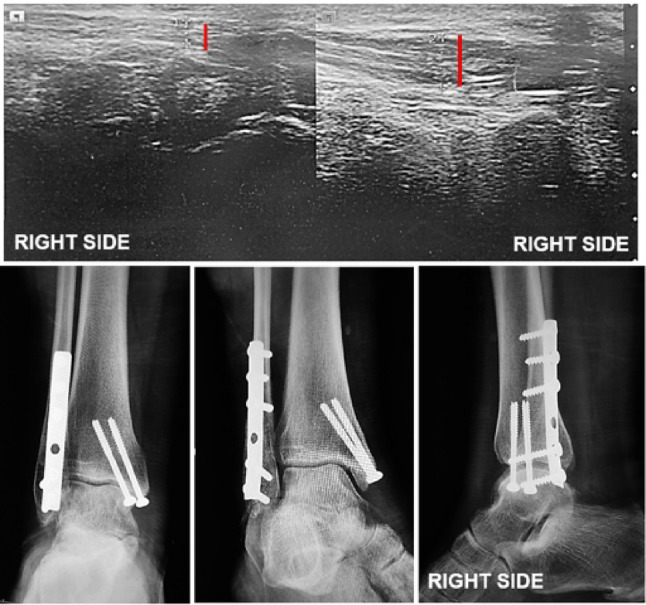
Ultrasound and plain radiographs of a right ankle fracture comparing the injured and contralateral tendons
